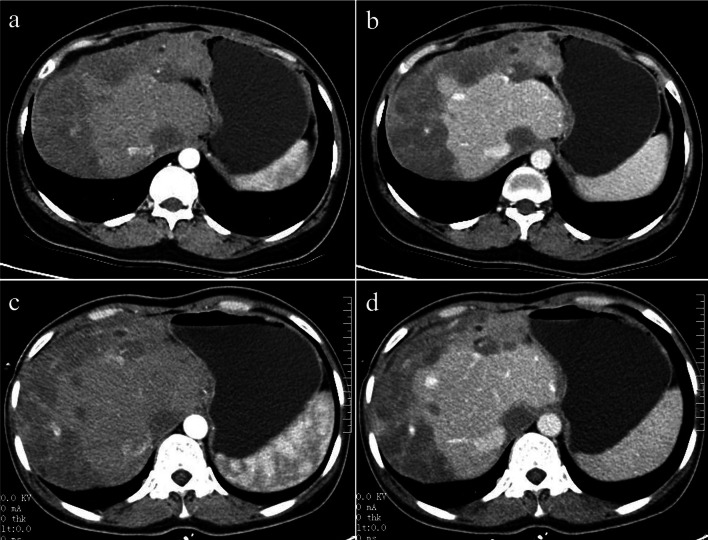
A 40-year-old female with diffuse nodular type HEH. Axial arterial and portal phase CT images (a, b) show multiple, diffuse nodules that lack obvious enhancement and confluent masses with strip-like enhancement located at the subcapsular region of the liver, accompanied by liver morphological changes and proportion imbalance. On follow-up CT performed 18 months later without any treatment, axial arterial and portal phase images (c, d) show no obvious changes in the lesions
